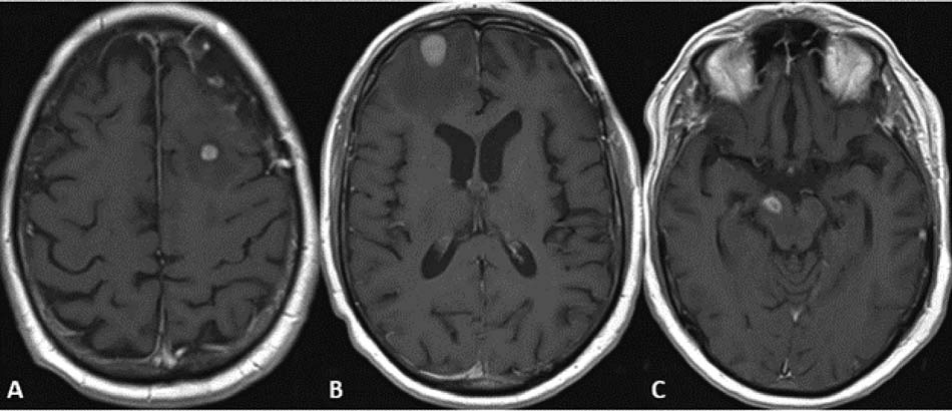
MRI scans indicating new metastatic lesions of the bilateral frontal lobes and right cerebral peduncle.
